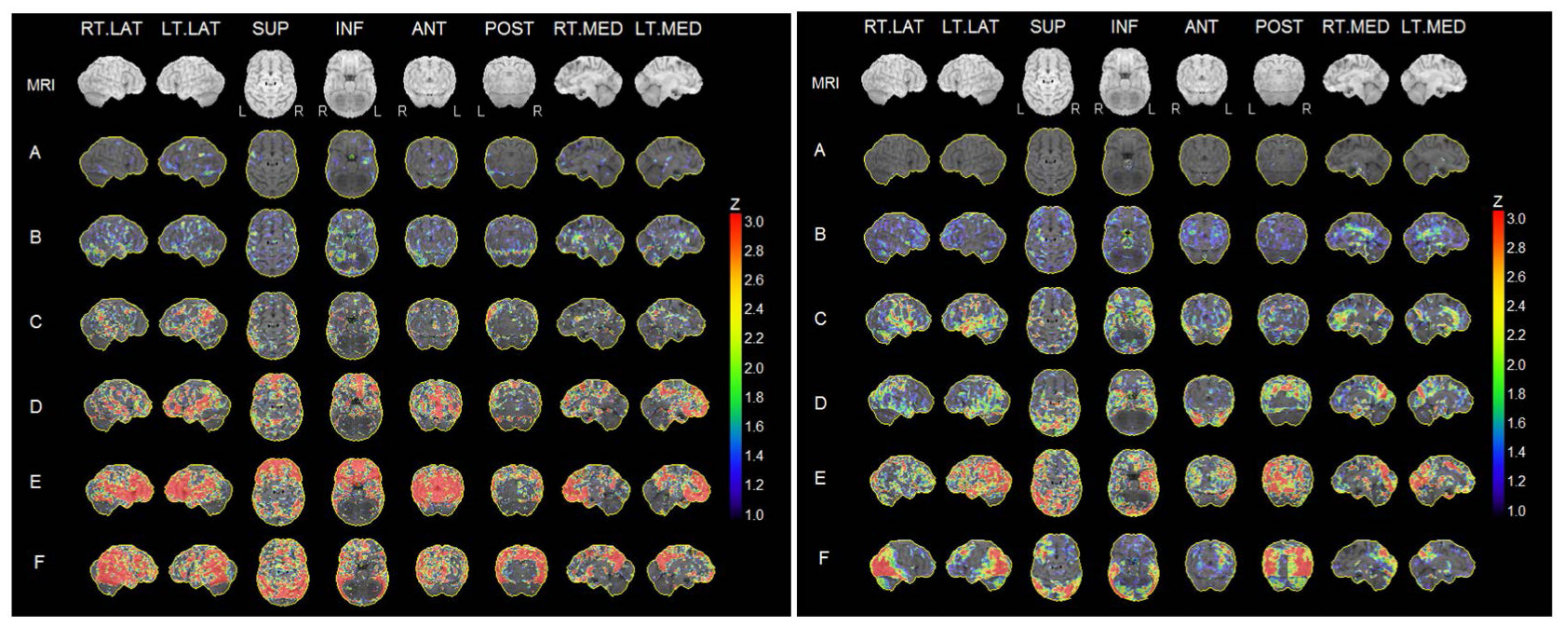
Left panel: Representative FDG-PET patterns in NL individuals: (A, B) FDG−; (C, D) mild hypometabolism of PCC a/o parieto-temporal cortex; (E, F) moderate-to-severe hypmetabolism of PCC a/o parieto- temporal cortex. Right panel: Representative PiB-PET patterns in NL individuals: (A, B) PiB−; (C, D) mild PiB uptakein PCC a/o parieto-temporal cortex; (E, F) moderate-to-severe PiB uptake in PCC a/o parieto- temporal cortex. 3D-SSP maps showing tracer uptake deviations relative to norms are displayed on a color- coded scale and shown on the right and left lateral, superior and inferior, anterior and posterior, right and left medial views of a standardized brain image.
